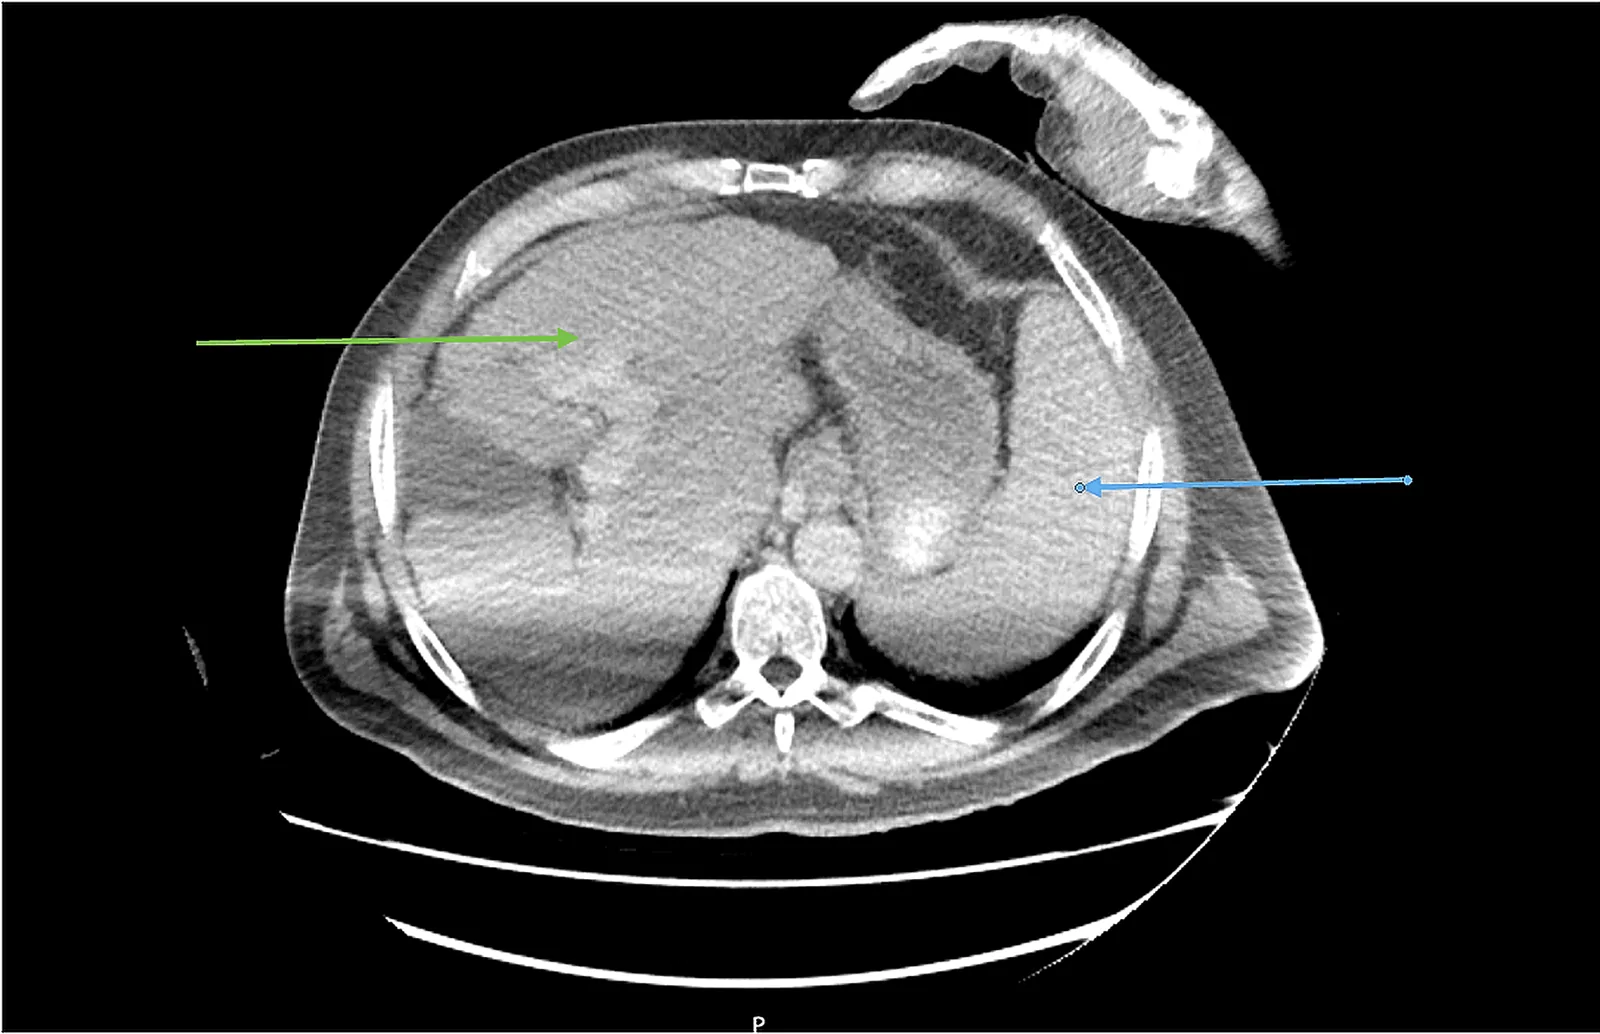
CT Abdomen and pelvis done on 16th October 2025 showing cirrhotic liver (marked with green arrow) with bulky spleen (marked with blue arrow).
